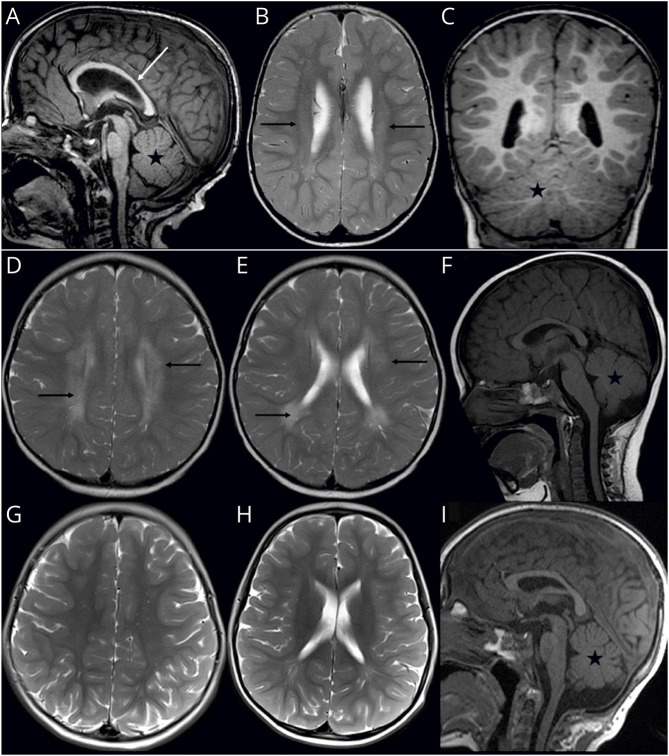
Brain MRI Findings Associated With KIF1C^Leu278Pro^ Patient 1 (A–C). Brain MRI at age 3 years and 2 months showed enlarged lateral ventricles, thin corpus callosum (A, white arrow), decreased amount of white matter, and mild symmetrical periventricular T2 signal hyperintensity (B, black arrows) presenting hypomyelination. Normal cerebellum (C, star) without pontocerebellar hypoplasia. Patient 2 (D–I). Brain MRI at age 1 year and 8 months showed periventricular T2-hyperintense white matter suggesting delayed myelination (D and E, black arrows), but at age 3 years and 8 months (G and H), myelination was normal. Normal cerebellum (F, I, star) without pontocerebellar hypoplasia.
